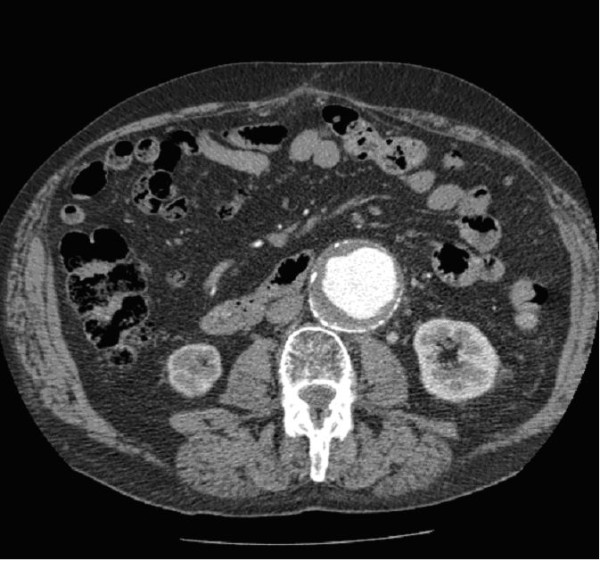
Computed tomography scan showing a densely calcified aorta measuring 5.9 cm.
